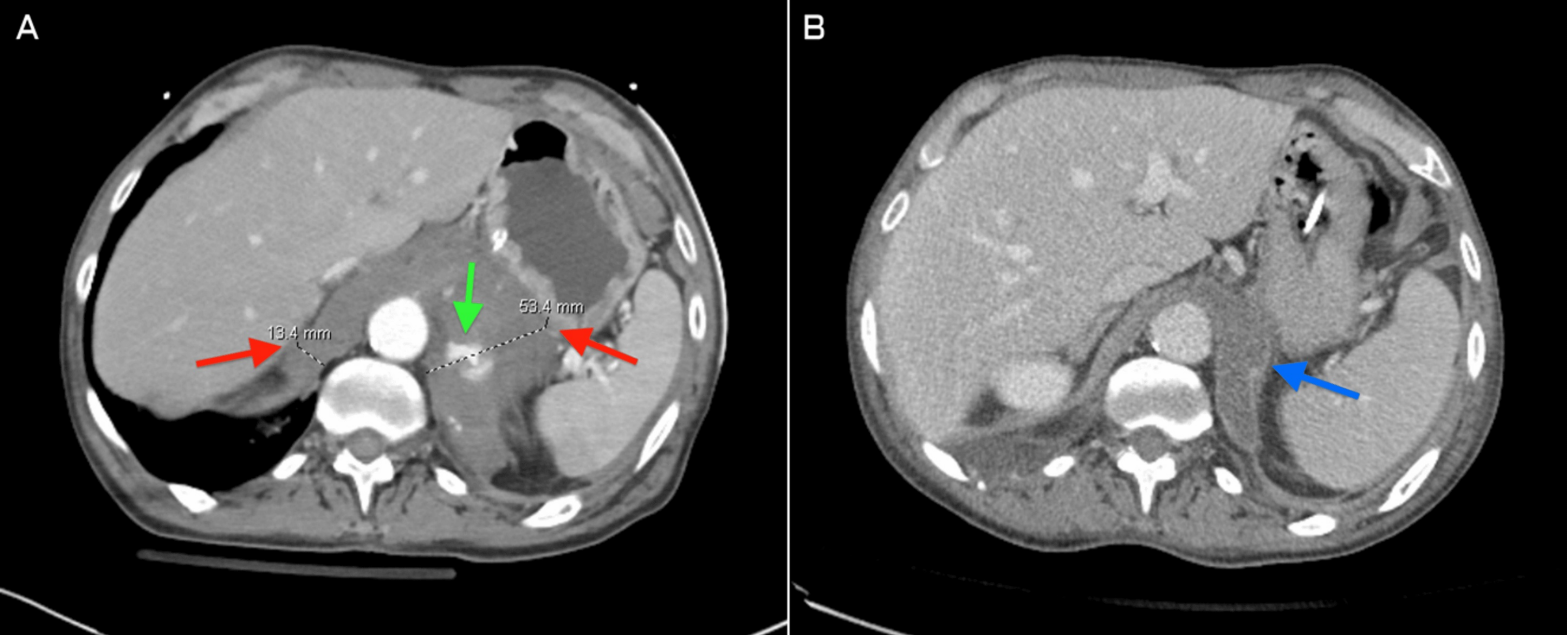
(A) Initial abdominal axial CT demonstrating active contrast extravasation (green arrow) and heterogenous thickening of the right and left diaphragmatic crura, measuring 13.4 mm and 53.4 mm, respectively (red arrows), consistent with hematoma within the right and left diaphragmatic crura. (B) 15-day follow-up. Abdominal axial contrast-enhanced CT shows near-complete resolution of the right diaphragmatic hematoma and significant reduction in size of the left diaphragmatic hematoma (blue arrow). CT: computed tomography
